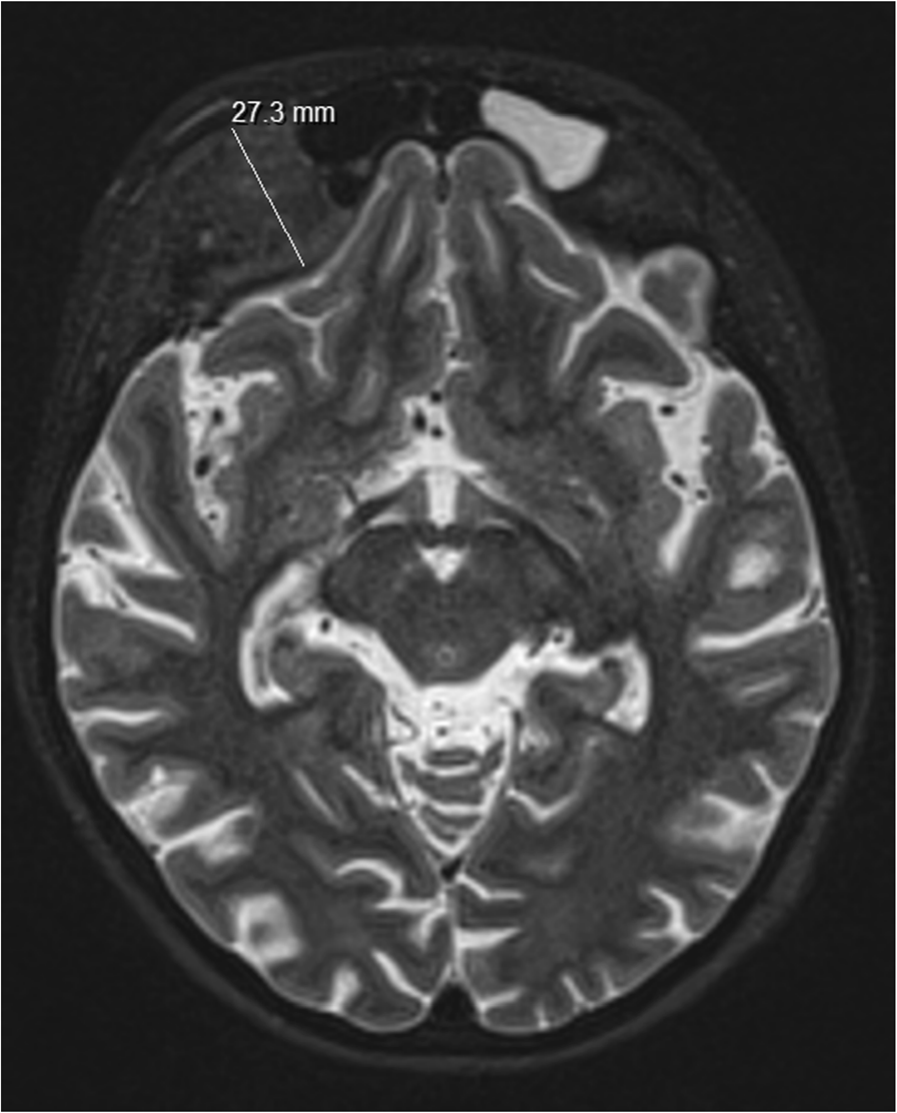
MRI of the patient‘s head. Measuring of hyperostosis of frontal bone on the right is shown by a white line
